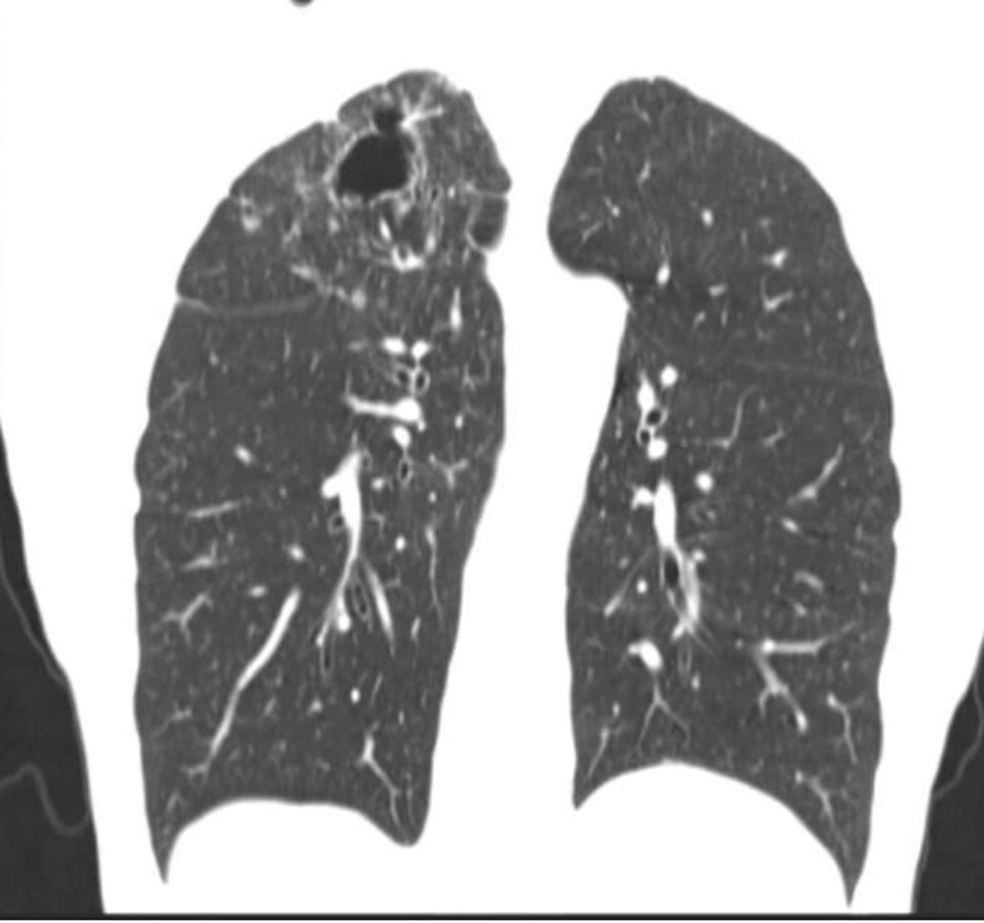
Repeat CT of the chest showing marked improvement in cavitary lesion with decreasing size and wall thickness in the right upper lobe CT: computed tomography
